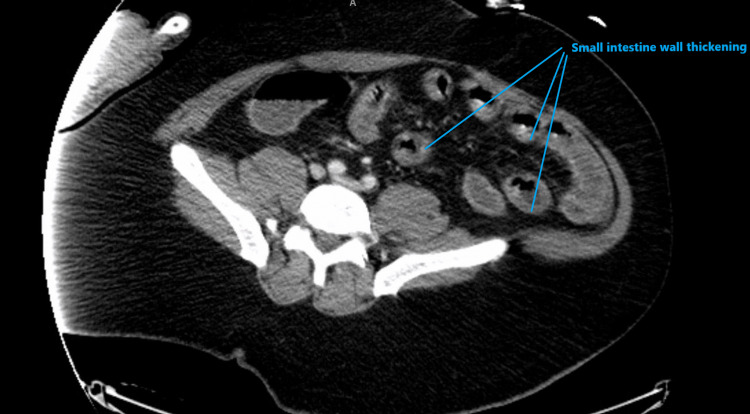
CT of the abdomen and pelvis with IV contrast showing the wall thickening of the small bowel IV: intravenous
